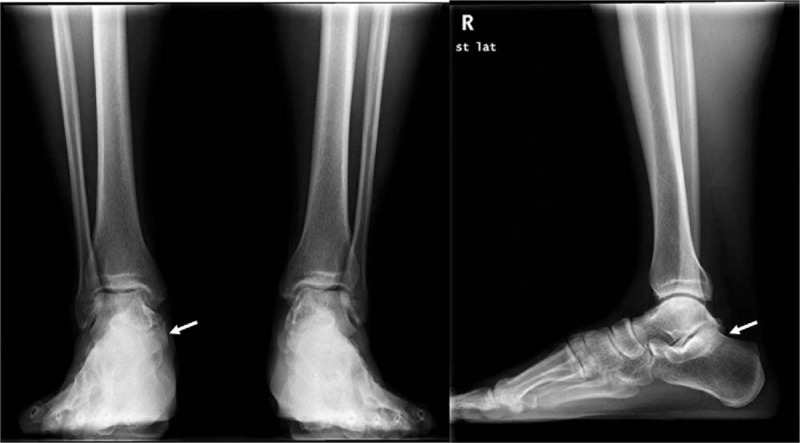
Preoperative plain standing anteroposterior (A) and lateral (B) radiographic views of the right ankle showing an accessory ossicle (arrow), which is located posterosuperomedially to the sustentaculum tali. The ossicle is the os sustentaculi.
